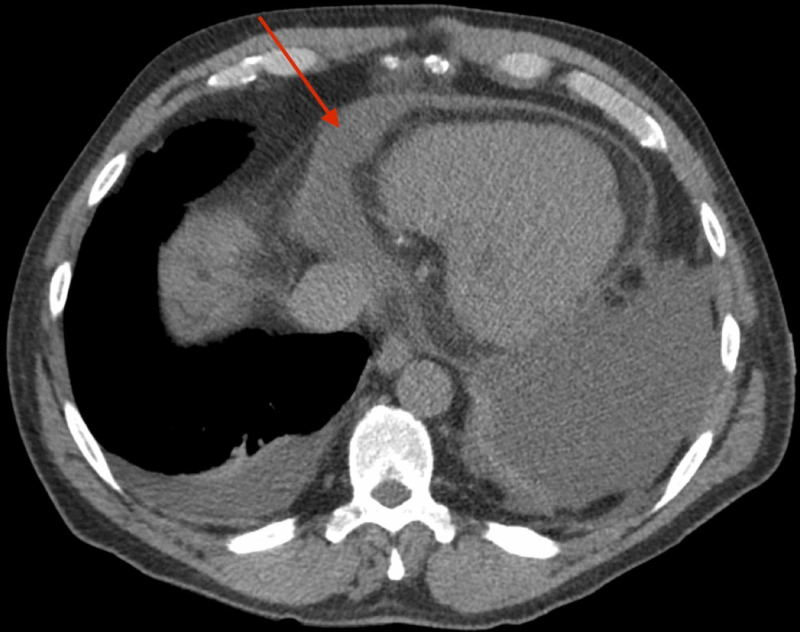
CT chest with contrast confirmed an interval decrease in the size of the pericardial effusion following catheter drainage
